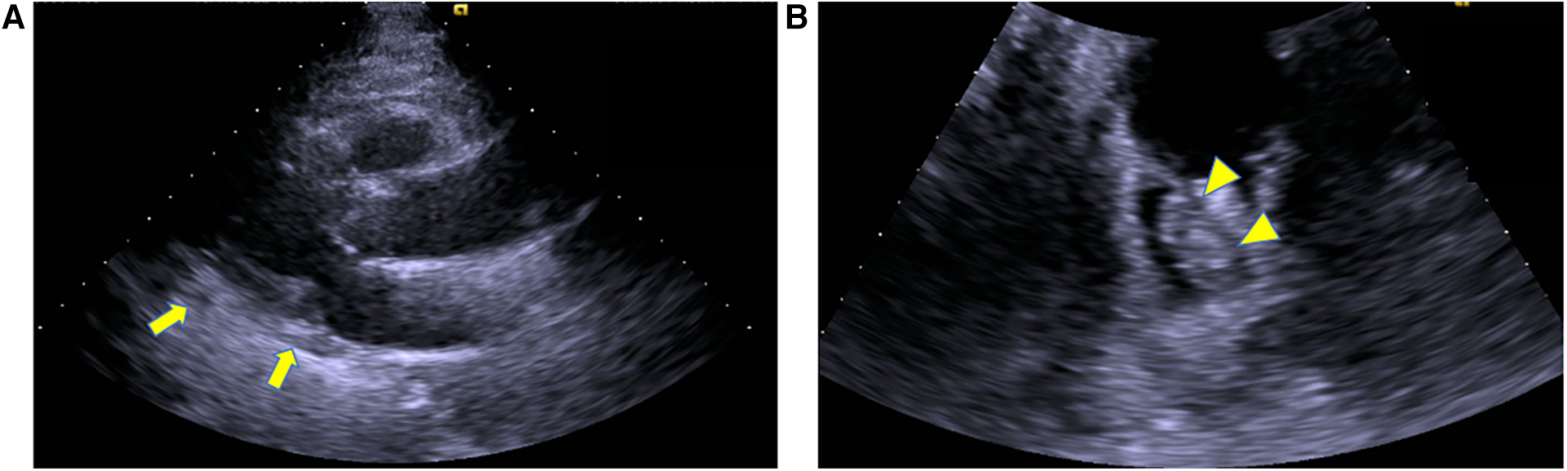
Postoperative echocardiography, (A) left ventricular long axis view, (B) 4 chamber view.echocardiography showed no pericardial effusion (arrow). It showed a floating mass extending from the left atrial orifice of the right pulmonary vein toward the atrial septum, which was considered a potential embolic source (arrowhead).
